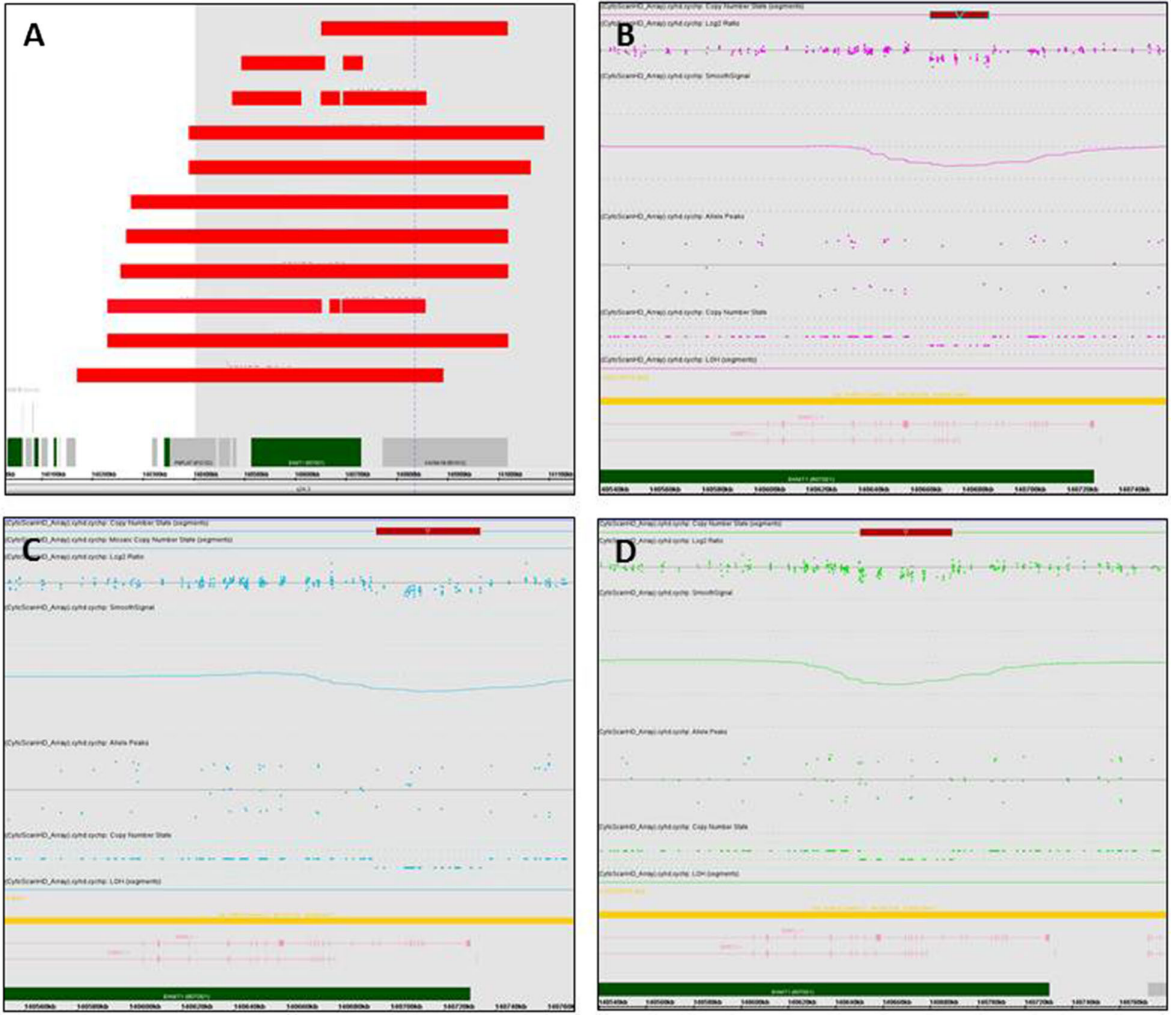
a The genomic locations of the 16 small (<1 Mb) pathogenic deletions involving the *EHMT1* gene. b. A 22-kb deletion (chr9:140,667,619-140,689,934) was found in 32-year-old female with intellectual disability. c A 40-kb deletion (chr9:140,694,541-140,734,178) was identified in a 5-year-old girl. d A 39-kb deletion (chr9:140,650,471-140,689,373) was discovered in a 1-year-old girl with developmental delay, speech and motor delay, white matter changes on MRI, and hypotonia
